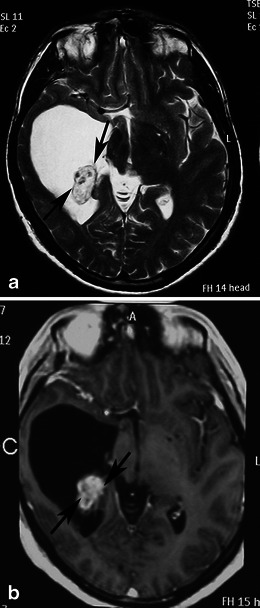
Classical appearance of a hemispheric pilocytic astrocytoma in a 45-year-old man. a An axial T2-weighted image demonstrates a hyperintense cystic component and a less hyperintense solid nodule within the lateral ventricle (arrows). b A contrast-enhanced axial T1-weighted image reveals intense enhancement of the solid nodule (arrows) and lack of enhancement of the cystic portion
